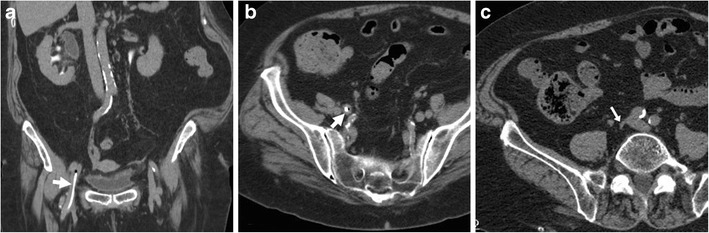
Long-term venous collapse following central venous catheterisation. On multidetector CT-urography (A, B) performed to investigate urosepsis in a 76-year-old woman, a right femoral CVC (thick arrows) is present. A year later, unenhanced CT image (C) incidentally showed very thin lumen of the ipsilateral common (arrow) and external iliac veins
